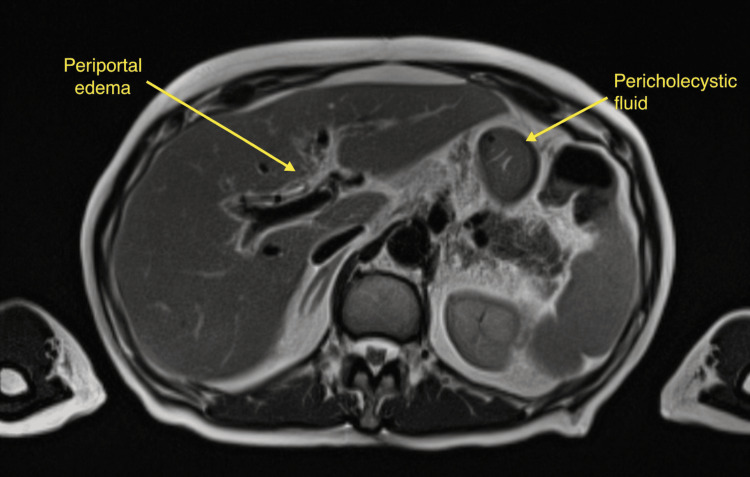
MRI/MRCP during acute illness. Axial T2-weighted MRI demonstrating hepatic heterogeneity with periportal edema and pericholecystic fluid without biliary ductal dilation or choledocholithiasis. These findings were initially concerning for biliary pathology but were ultimately attributed to disseminated HSV-2 infection with hepatic involvement. *Abbreviations*: MRI, magnetic resonance imaging; MRCP, magnetic resonance cholangiopancreatography; HSV-2, herpes simplex virus type 2.
